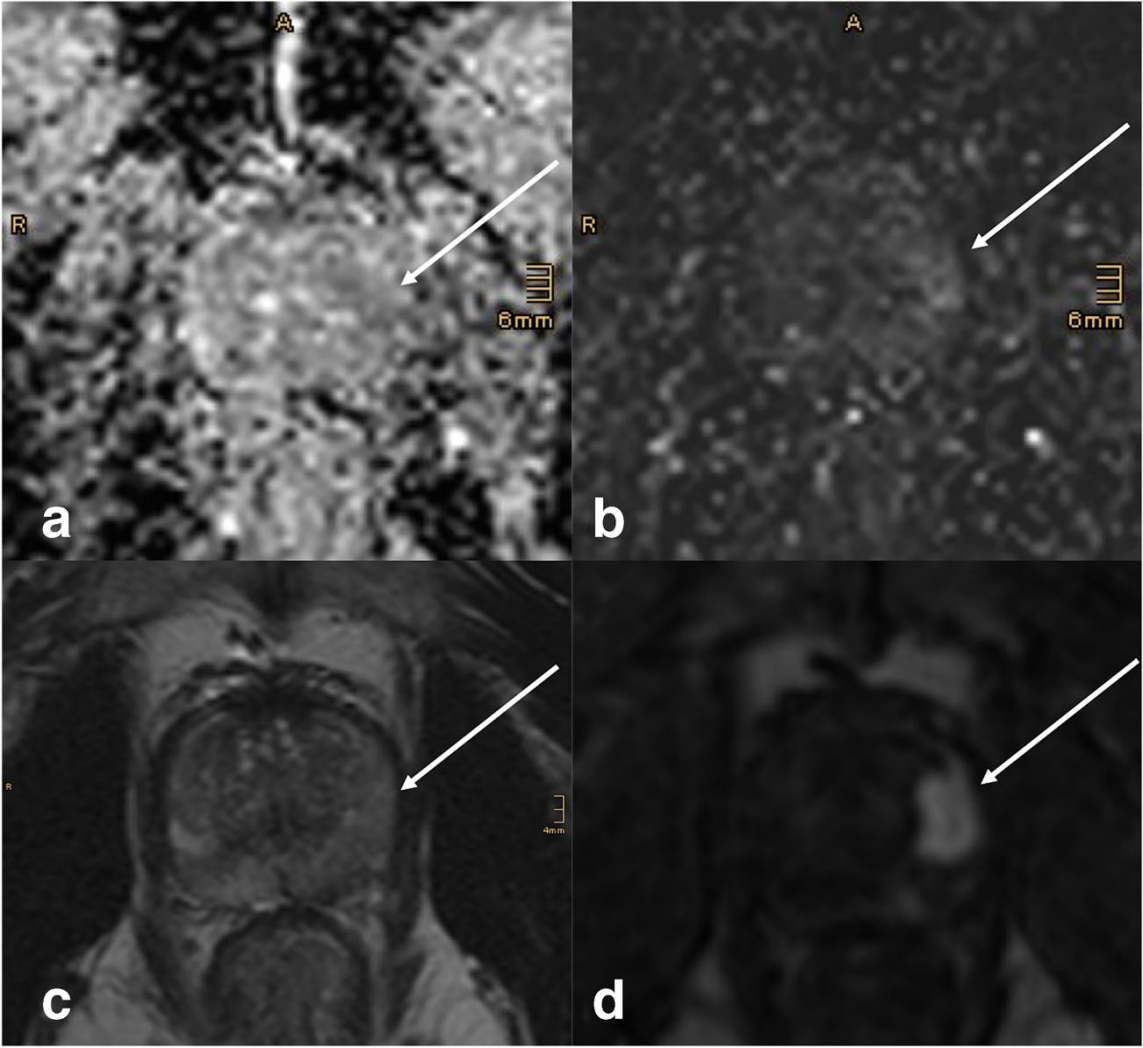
51-year-old man with a biopsy-proven ISUP 5 invasive prostate cancer visible on multiparametric MRI (white arrows). The lesion measures > 15 mm and shows only mild changes on DWI (a ADC map, b high *b*-value) and T2w (c). With poor visibility, the lesion might have been missed on biparametric MRI. Lesion visibility, however, is excellent on early DCE (d), and allows for lesion detection, and subsequent grading according to PI-RADS criteria
